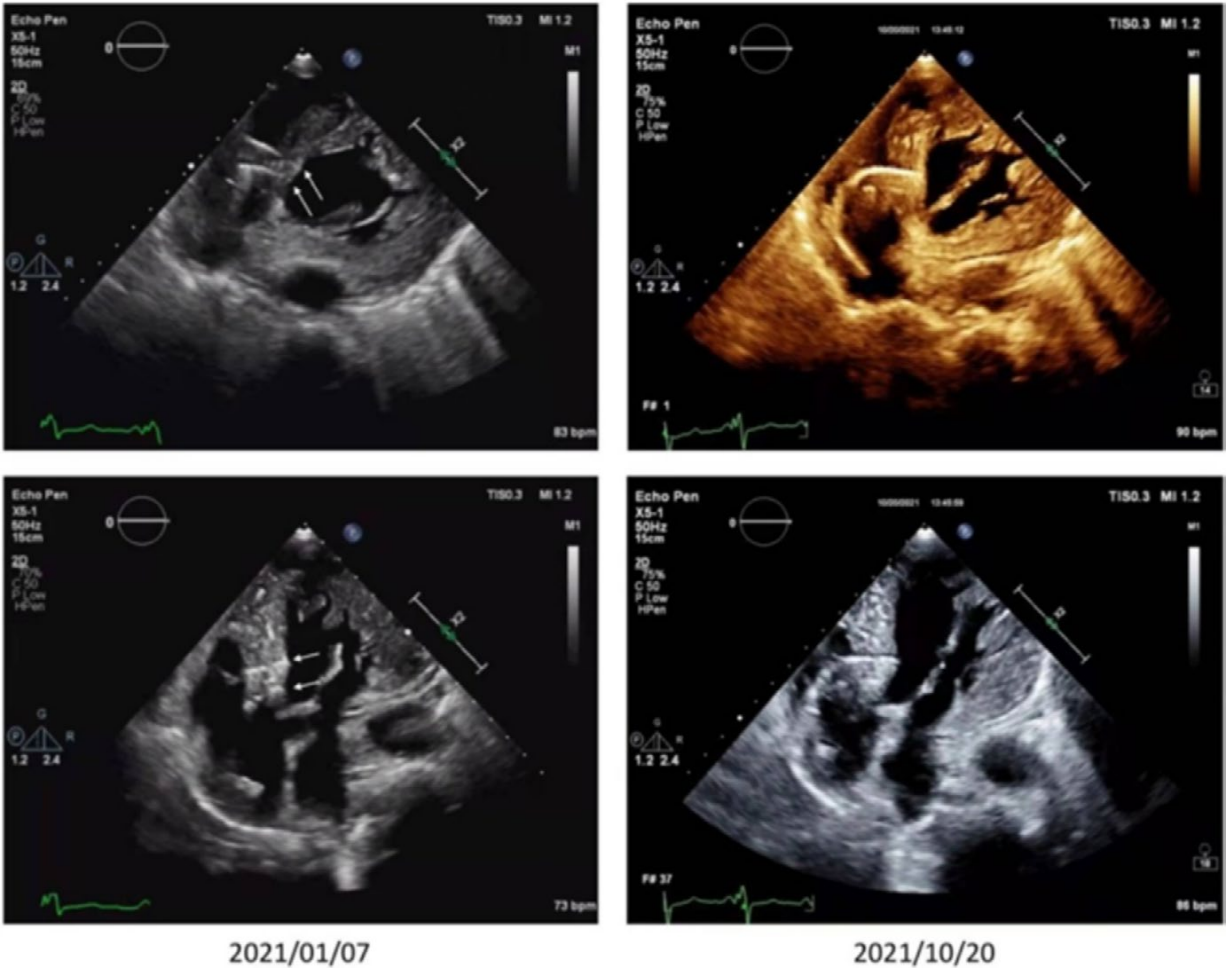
Echocardiograms showing lead positions 3 months and 1 year after CRT implantation. The interventricular septum thickened, but the positions of the leads remained stable.
